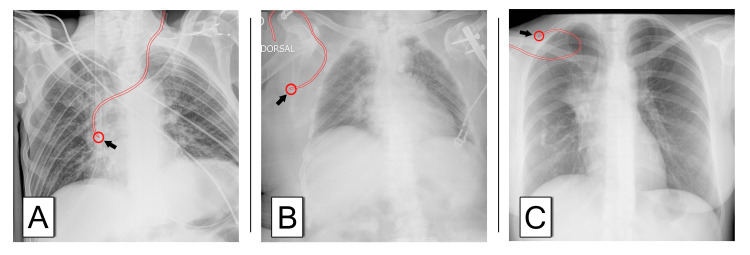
Post-procedure chest radiographs. Examples of post-procedure chest radiograph (CXR) recorded during the study period. (A) Indication: parenteral nutrition, duration: 84 days, removal reason: missing; (B) Indication: absence of IV access, duration: 10 days, removal reason: death; (C) Indication: chemotherapy, duration: two days, removal reason: end of need.
